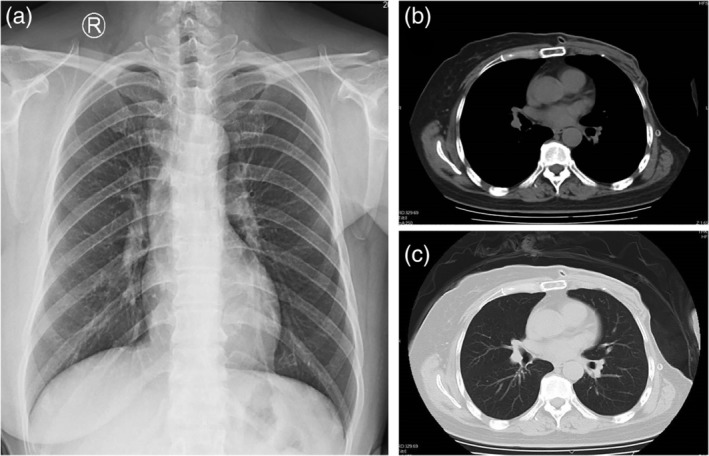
Digital radiography (D) and computed tomographic (CT) examination of the chest after surgery (a) DR of the chest, (b) Mediastinal window view of the breast and (c) Lung window view of the breast.
